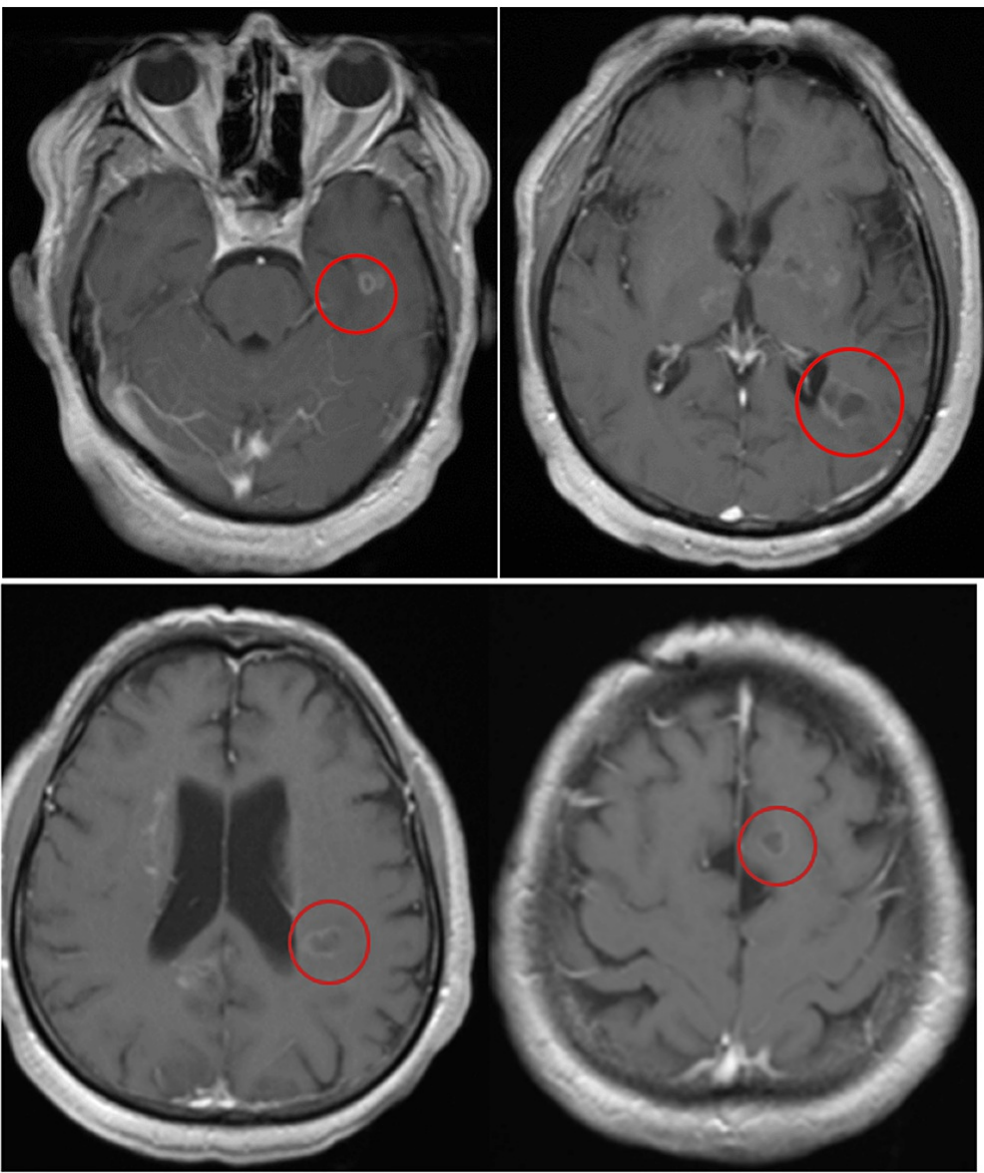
MRI brain T1 sequence showed four ring-shaped post-contrast enhanced lesions (red circles).
